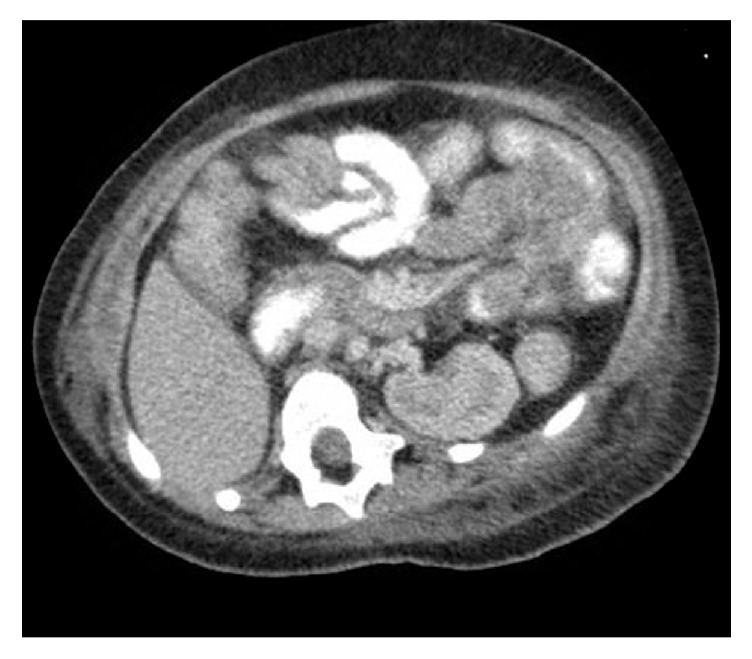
Abdominal CT scan showing solitary left kidney with kidney cyst and nephrocalcinosis.
